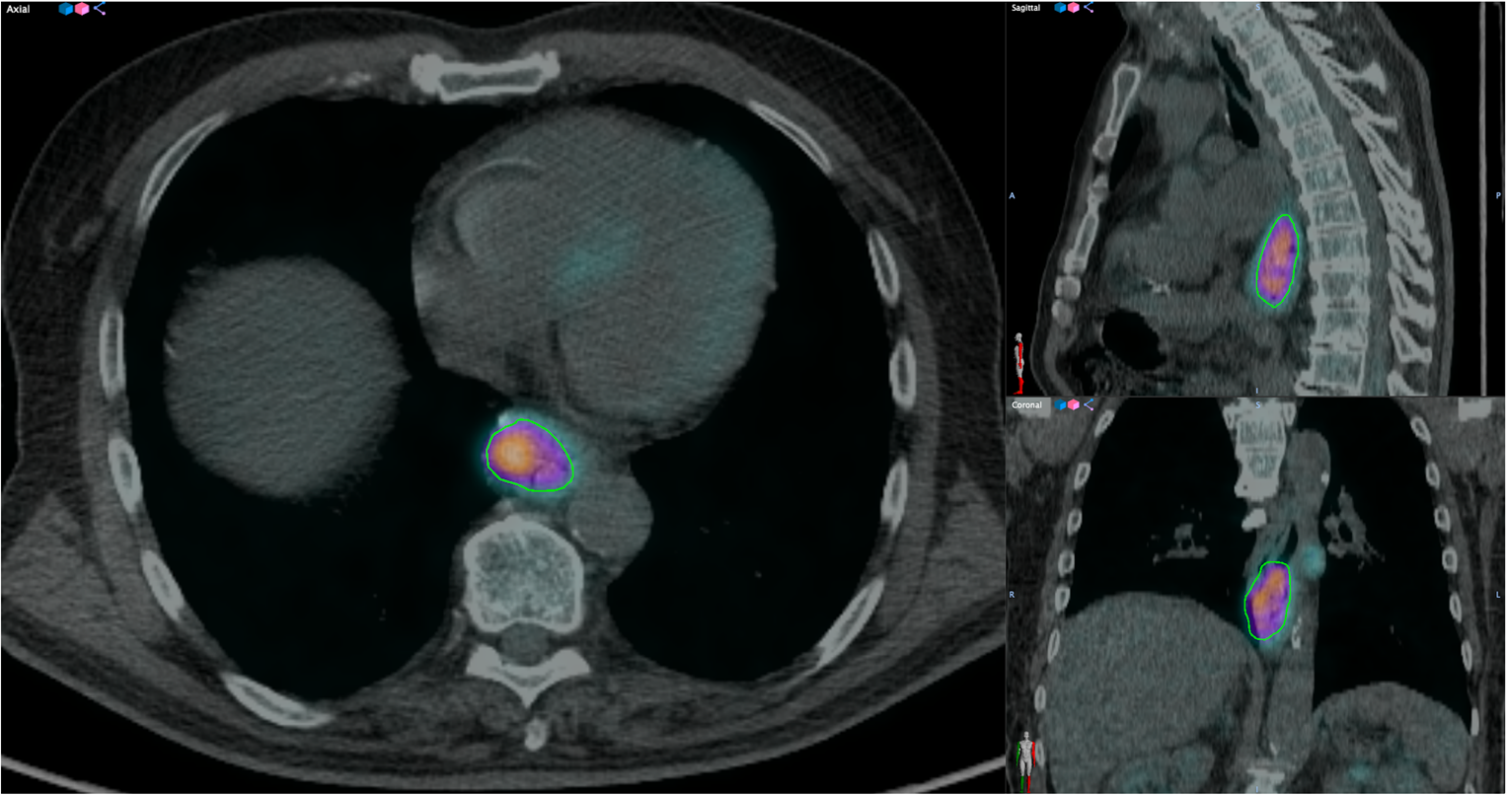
Fused axial, sagittal and coronal FDG-PET/CT images of an oesophageal tumour outlined with ATLAAS segmentation tool
